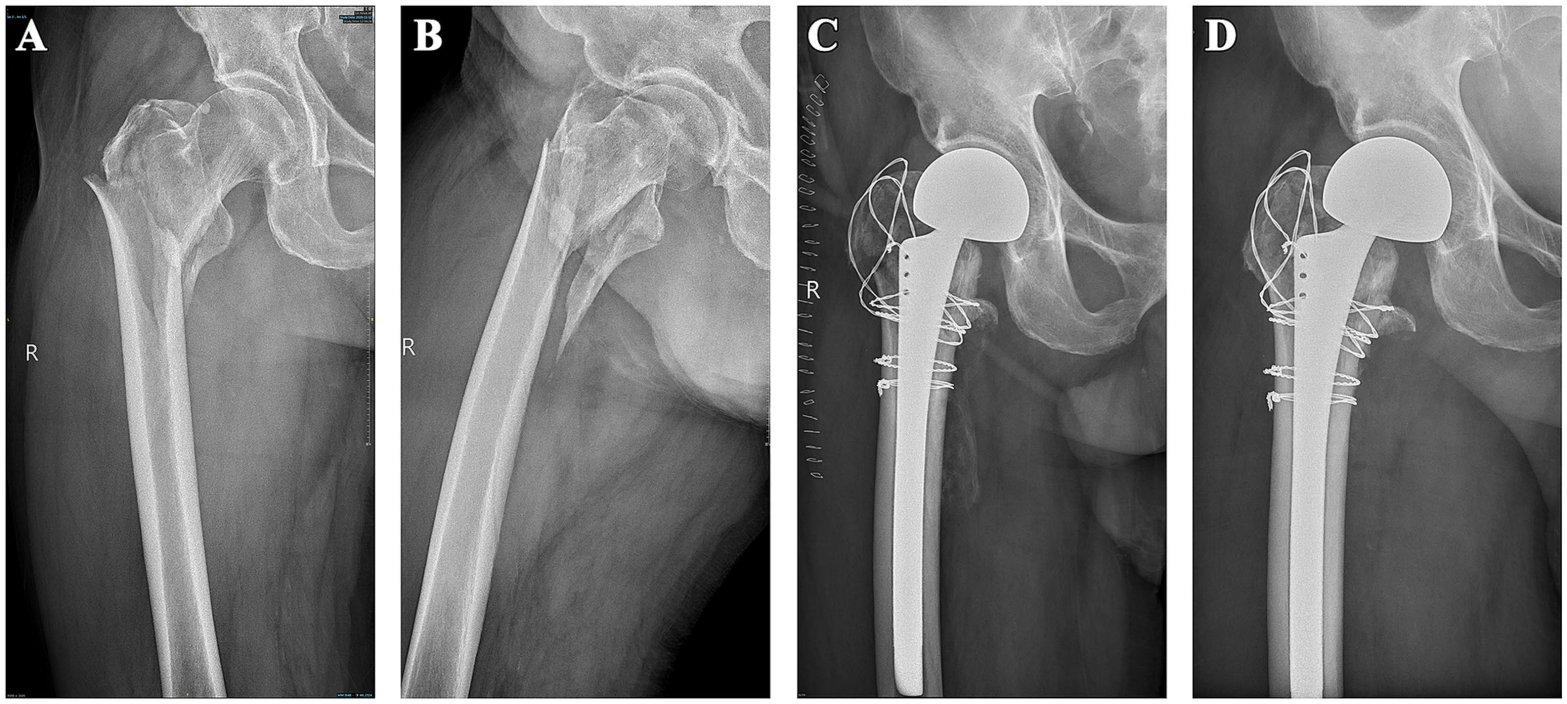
Characteristics of a typical patient’s case. (A,B) A preoperative frontal view of the lateral view of the upper and middle right femur reveal a comminuted fracture of the right femoral trochanter with obvious displacement. (C) X-ray on the first day after operation. (D) X-ray shows fracture healing and the position of the artificial joint is good 1 year after operation.
